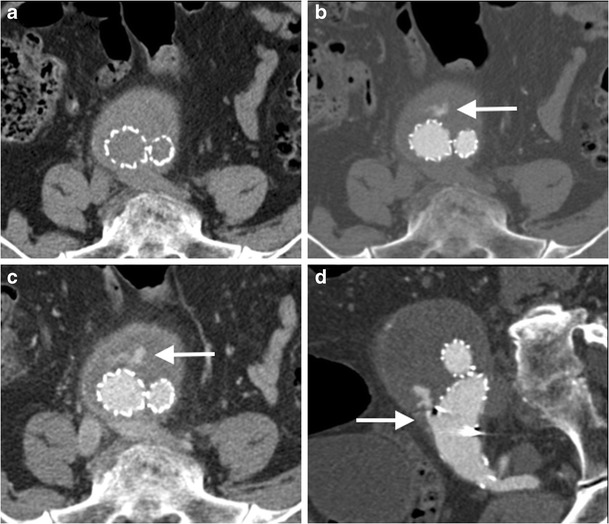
Selected images from a CT angiogram in a 55-year-old man undergoing imaging surveillance post abdominal aortic aneurysm endovascular stent graft repair (EVAR). Axial non-contrast (a), arterial phase (b) and 70 s delayed phase (c) images demonstrate iodinated contrast material within the excluded aneurysm sac (*arrow*) consistent with an endoleak. Sagittal oblique MPR of the right common iliac artery (d) demonstrates the endoleak arising from the distal insertion point of the right iliac limb of the EVAR consistent with a type 1b endoleak
